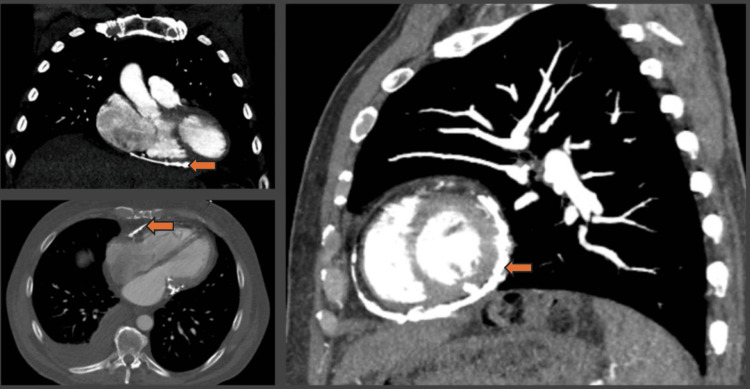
Chest CT demonstrating extensive pericardial calcifications (arrows), raising clinical suspicion for CP. CT: computed tomography; CP: constrictive pericarditis.
